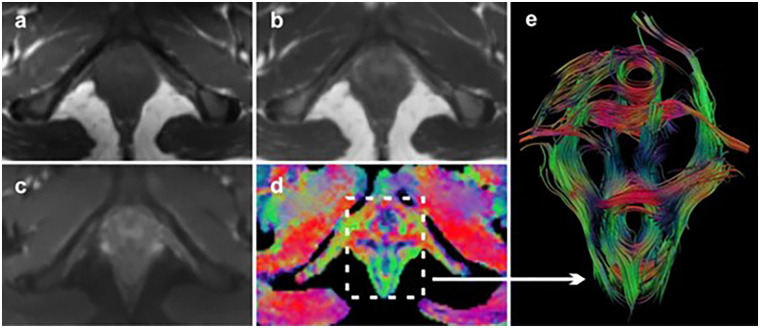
Example of pelvic floor muscle DTI fibre tracking representation. Axial T1-weighted image (a), T2-weighted image (b), b = 0 image (c) and corresponding FA map (d) With the application of whole volume seeding, present three dimensional (3D) fibre trajectories provide a comprehensive overview of the complex pelvic floor anatomy (caudal view) (e) Source: Reprinted from European Radiology (72). Copyright 2025, with Permission obtained from Springer Nature (License No. 6065941156804).
